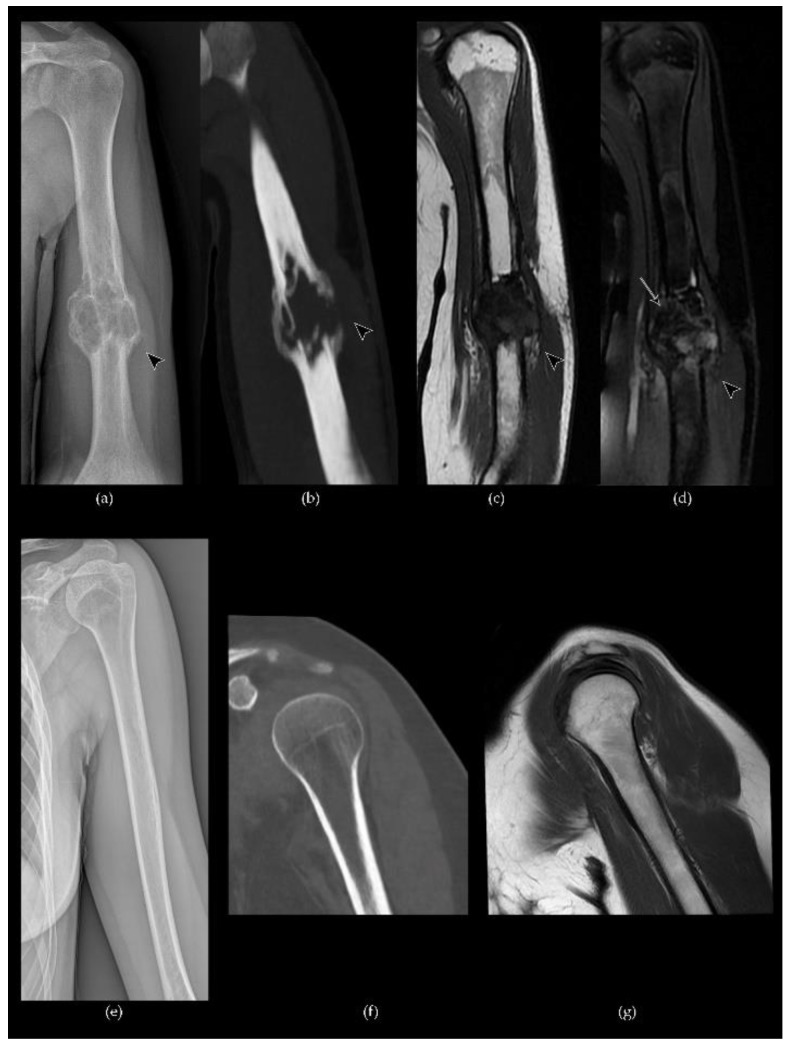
A case of GCTB of the left humerus diaphysis (a–d, arrowhead) compared to normal bone (e–g): (a) conventional radiography shows an osteolytic alteration of the bone with a pluri-chambered appearance in relation to the cystic components; (b) a coronal CT Maximum Intensity Projection (MIP) shows the erosion of the cortical bone with loss of the classic trabeculation and pathological fracture associated; (c) on a coronal T1-W FSE MR image, the lesion demonstrates inhomogeneous low signal intensity with a pathological involvement of the adjacent soft tissues; (d) a coronal fat-suppressed T2-W FSE MR image shows heterogenous signal intensity, with cystic components characterized by focal hyperintensity and hemosiderin deposits (arrow); (e–g) conventional radiography, CT and T1-W FSE MR images of normal bone findings.
